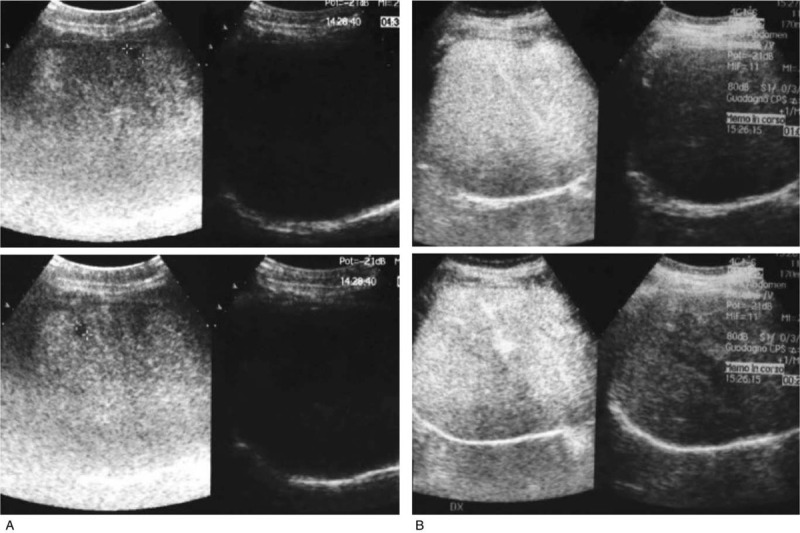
Liver ultrasound before (A) and after (B) 39 months of everolimus plus exemestane.
